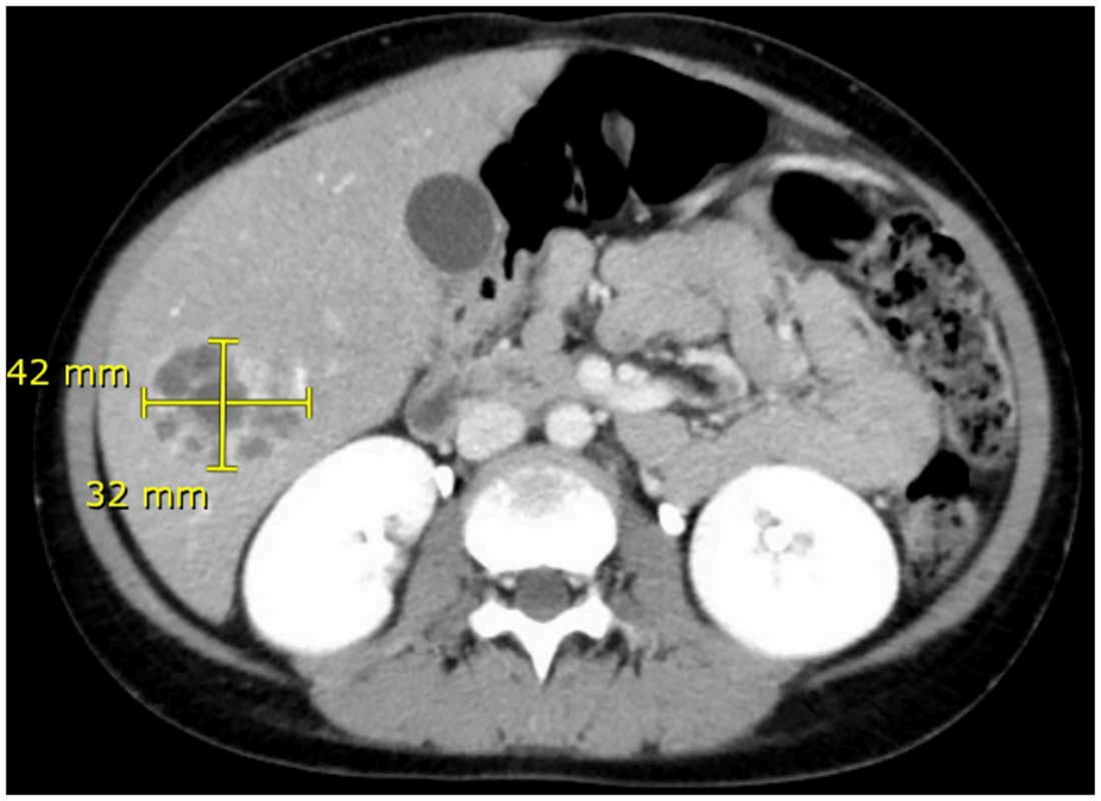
Cross-sectional abdominal CT showing 3.2 × 4.2 cm multilocular cystic lesion in liver segment 6. CT = computed tomography.
